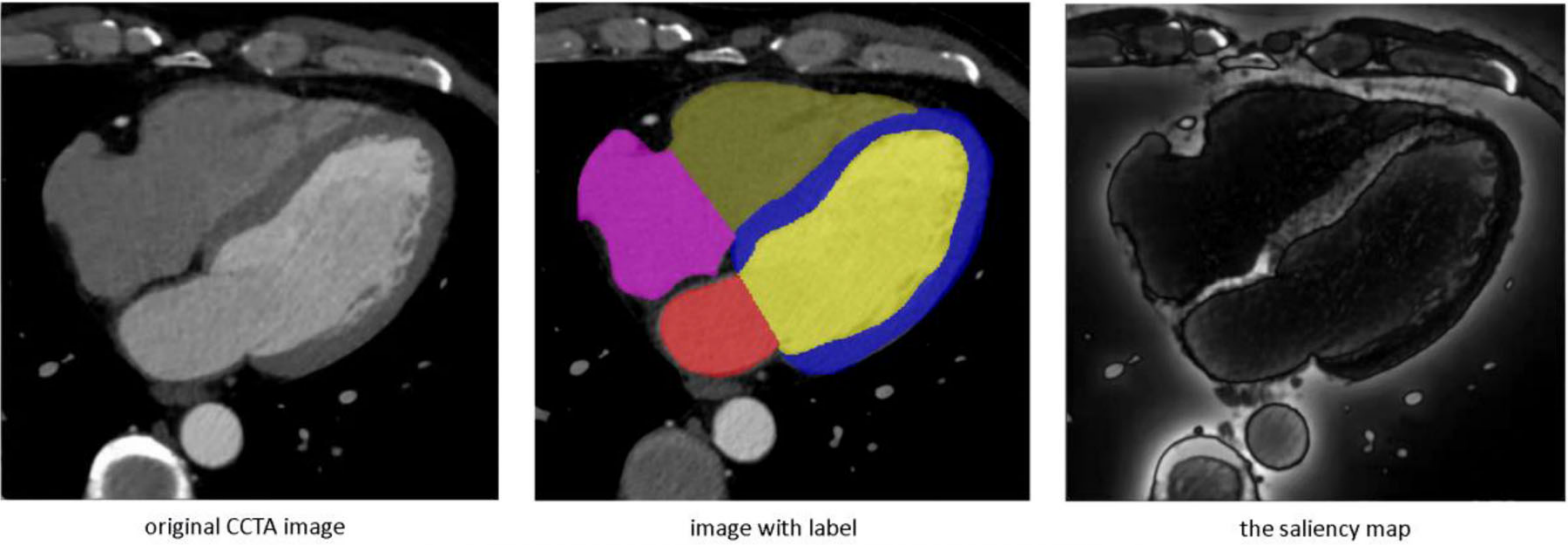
Compare and display the original coronary CT angiography (CCTA) image, the labeled image and the saliency map. From this picture, the addition of the saliency map improved the tissue contrast of the organs in the original CCTA image and also grasped more information about the boundaries. It improved the clarity of the CCTA image boundaries, which played a important role in the following segmentation
